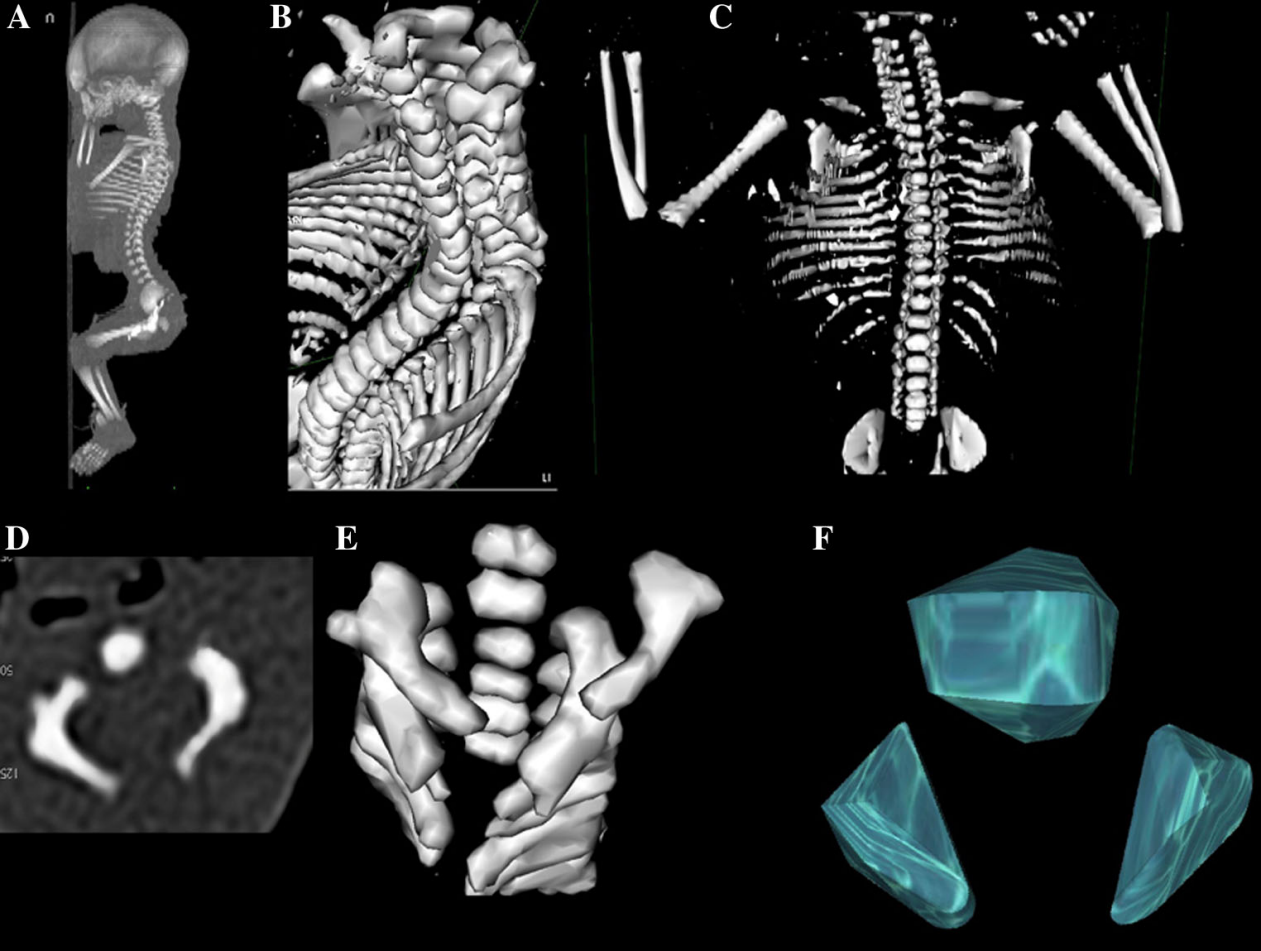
CT of a female fetus aged 23 weeks (a) recorded in DICOM formats and assessed by Osirix 3.9 in horizontal (b) and frontal (c) planes, with the transverse view of cervical vertebrae (d), reconstruction of the atlas and axis (e), and the body and neural arches of axis (f)
